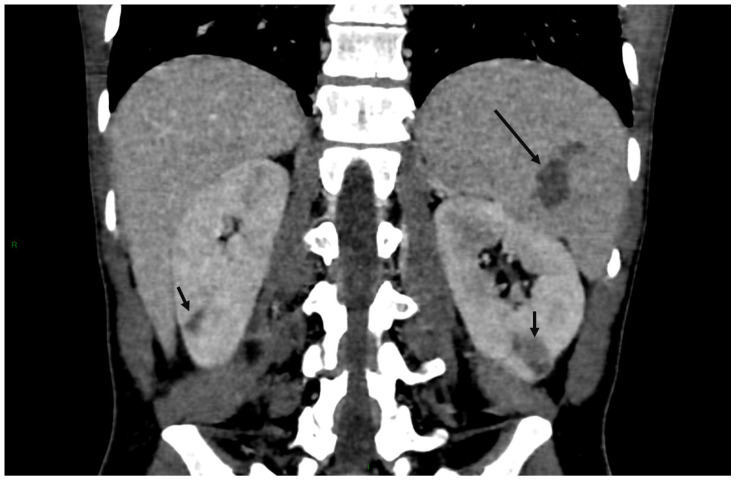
Infarcts of the spleen and both kidneys in a *Streptococcus oralis* infectious endocarditis complicated by a hepatic infectious aneurysm. Abdominal CT scan without contrast shows both splenic infarction (long arrow) and infarcts of right and left kidneys (short arrows).
